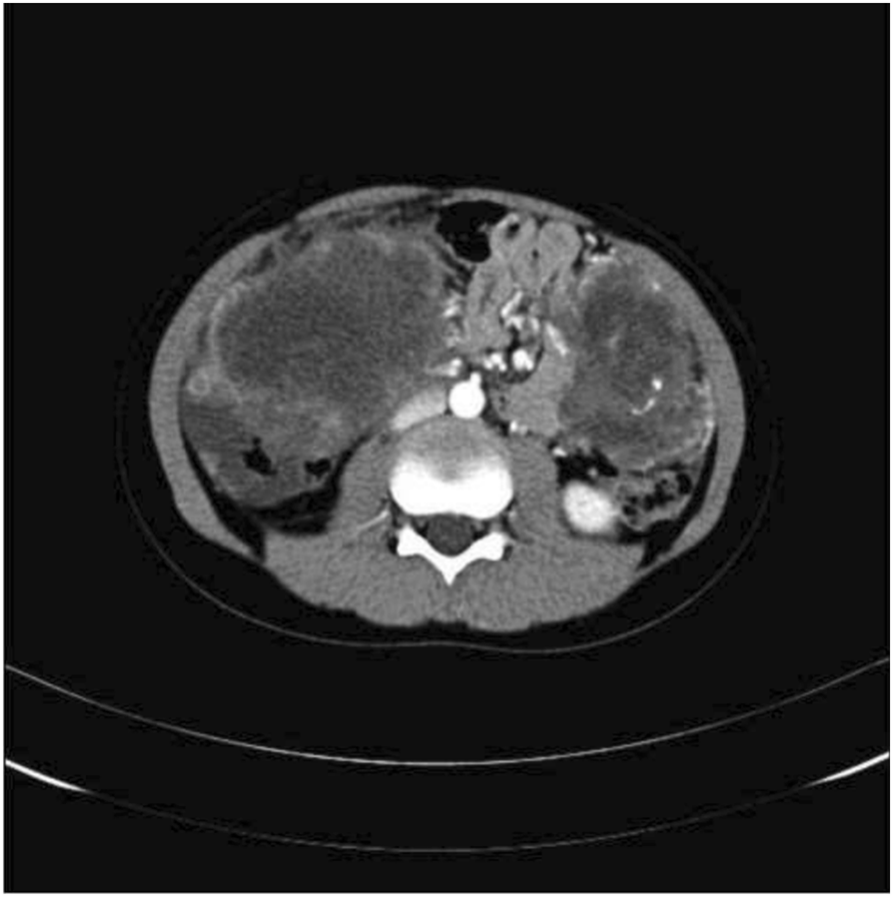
Enhanced CT image in the arterial phase of the mass in the right liver and abdominal cavity.
